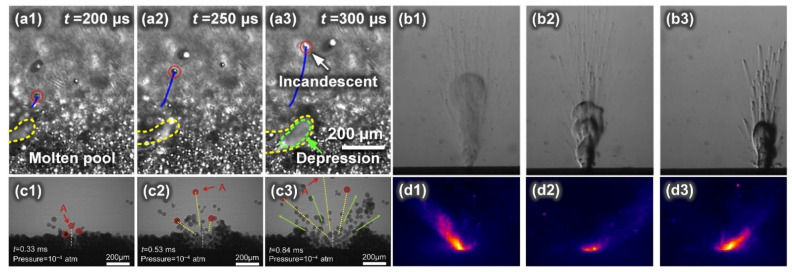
Characteristics obtained from different in situ detection techniques: (a1–a3) time series snapshots taken by visible light high-speed camera (Reprinted with permission from Ref. [34]. Copyright 2019 Elsevier B.V.); (b1–b3) high-speed schlieren images during single track scans (Reprinted with permission from Ref. [35]. Copyright 2018 Springer Nature.); (c1–c3) dynamic X-ray images showing powder motion, A is the ejected powder (Reprinted with permission from Ref. [36]. Copyright 2018 Elsevier B.V.); (d1–d3) three consecutive frames of an infrared video acquired during L-PBF (Reprinted with permission from Ref. [37]. Copyright 2018 Elsevier B.V.).
